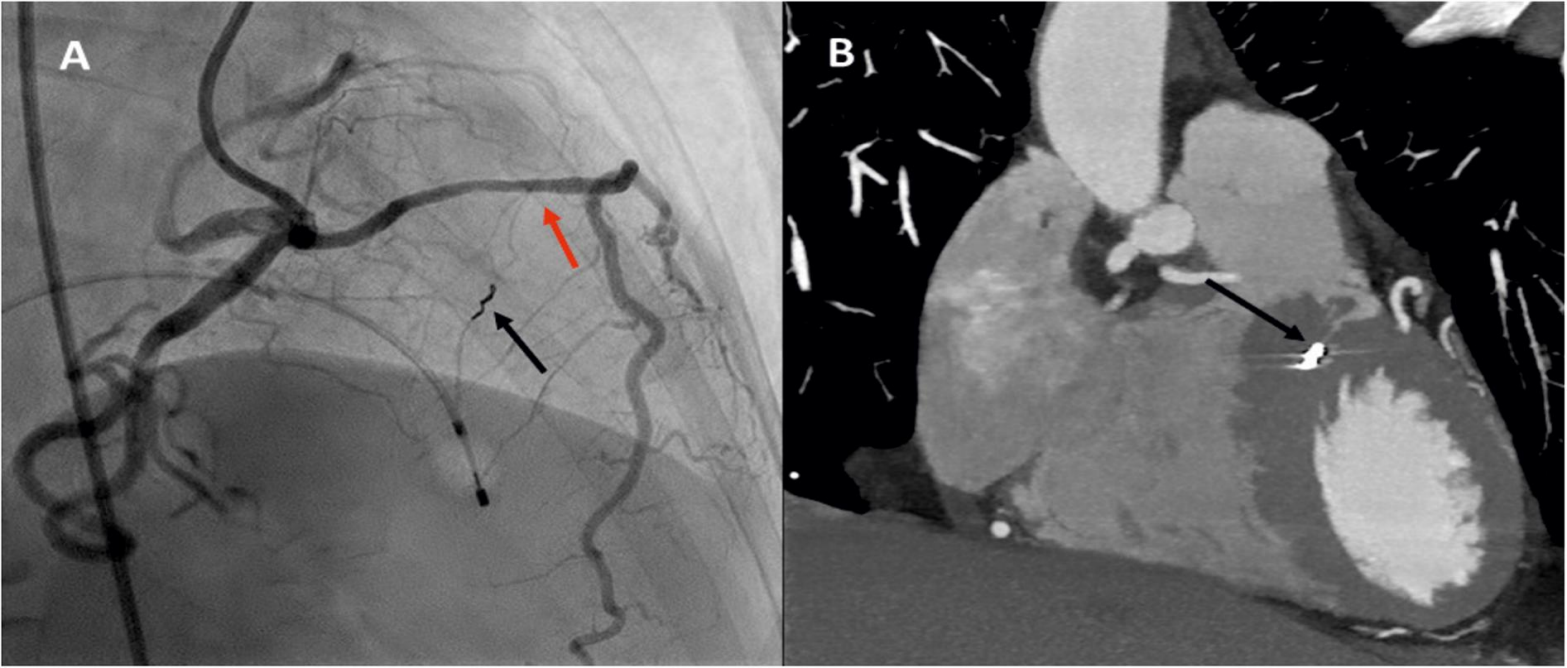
Invasive coronary angiography (A) and multiplanar reconstruction cardiac CT (B) with microcoil in septal branch acute take-off (red arrow) and microcoil (black arrow) positioned in the largest septal branch—figure 2A; micro coil in septal branch (black arrow)—figure 2B.
